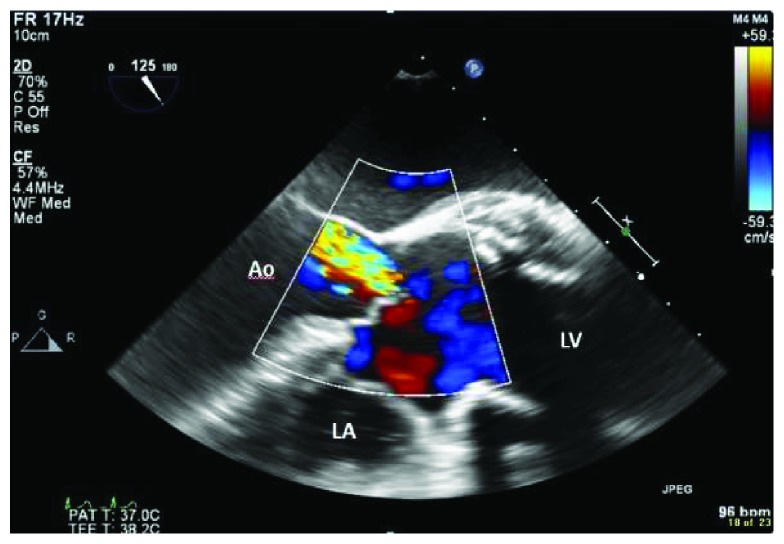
Mid-esophageal long-axis view of the heart in the transesophageal echocardiogram. White arrow shows periaortic abscess extending to the root of the aorta.
